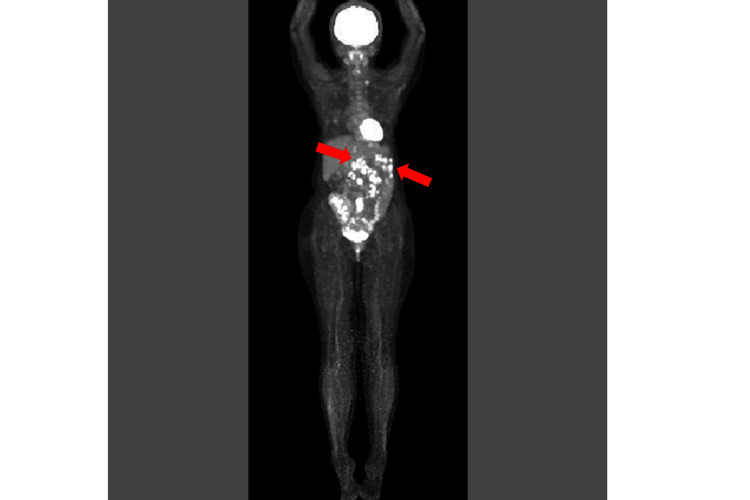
FDG-PET/CT at Initial Diagnosis Fluorodeoxyglucose positron emission tomography/computed tomography (FDG-PET/CT) demonstrates multiple hypermetabolic lymph nodes above and below the diaphragm, consistent with disseminated lymphoproliferative disease. A representative lesion is indicated by an arrow.
